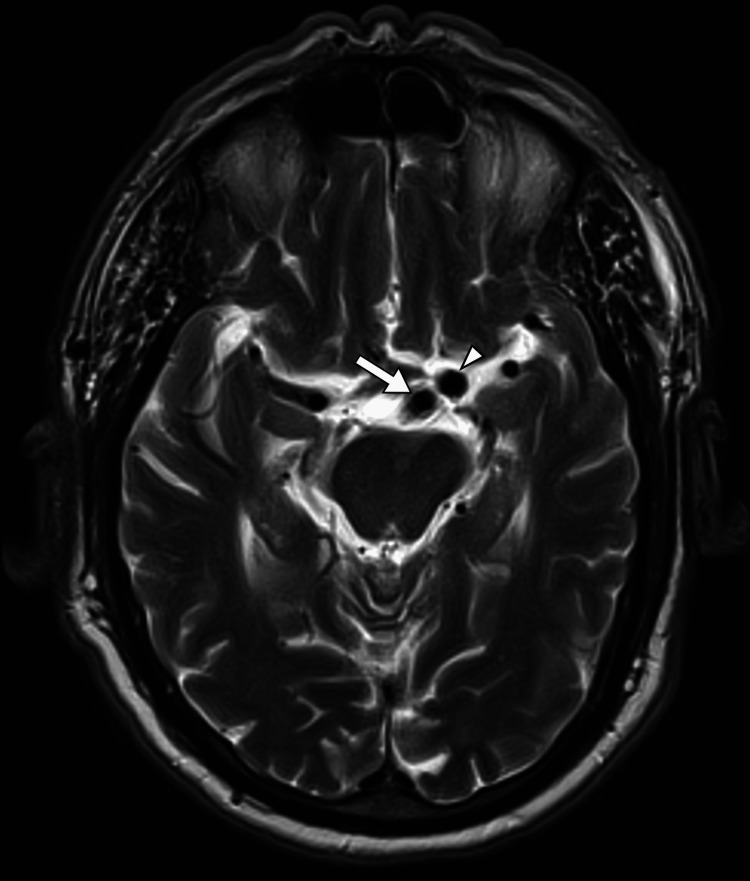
An axial T2-weighted image of the patient's brain MRI shows abnormal dilatation of the basilar artery (arrow), with its tip in the suprasellar cistern adjacent to the left middle cerebral artery (arrowhead).
